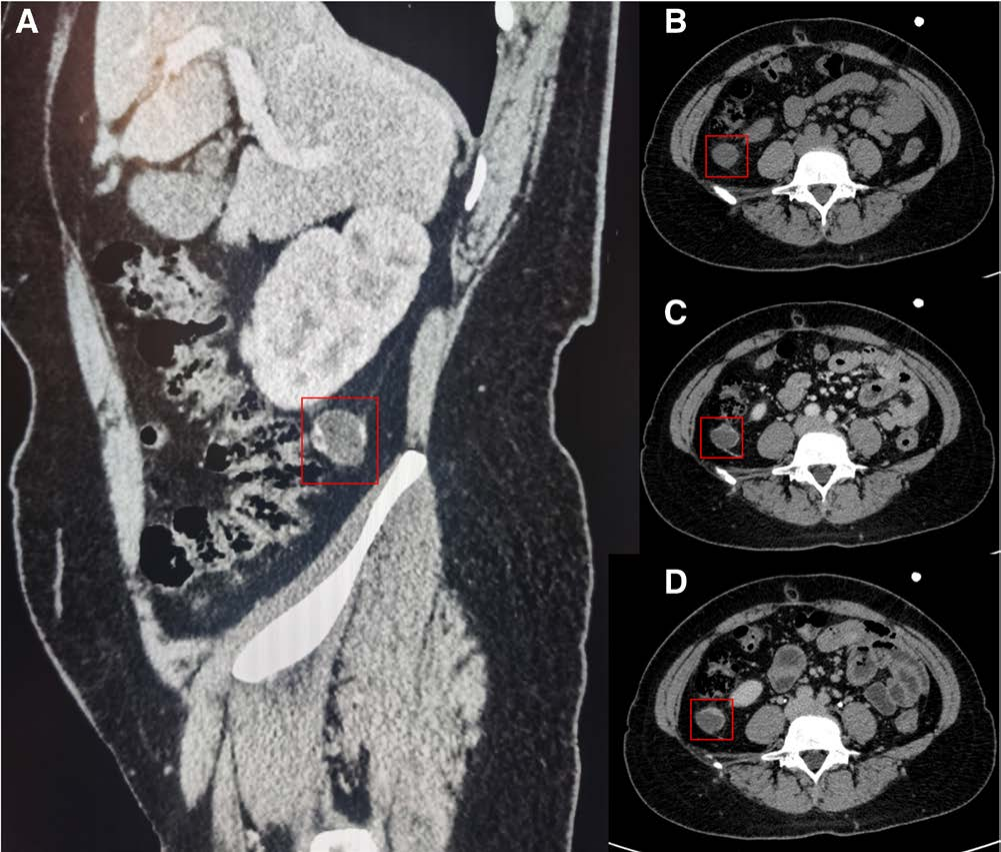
The abdominal CT scan (A) revealed a low-density cystic lesion measuring 2.8 cm × 2.4 cm in the perirenal space, located posterior to the lower pole of the right kidney. Multi-phase enhancement of the abdomen and pelvis (B–D) demonstrated significant enhancement of the cyst wall, which exhibited uneven thickness, along with the presence of cords surrounding the cyst wall.
